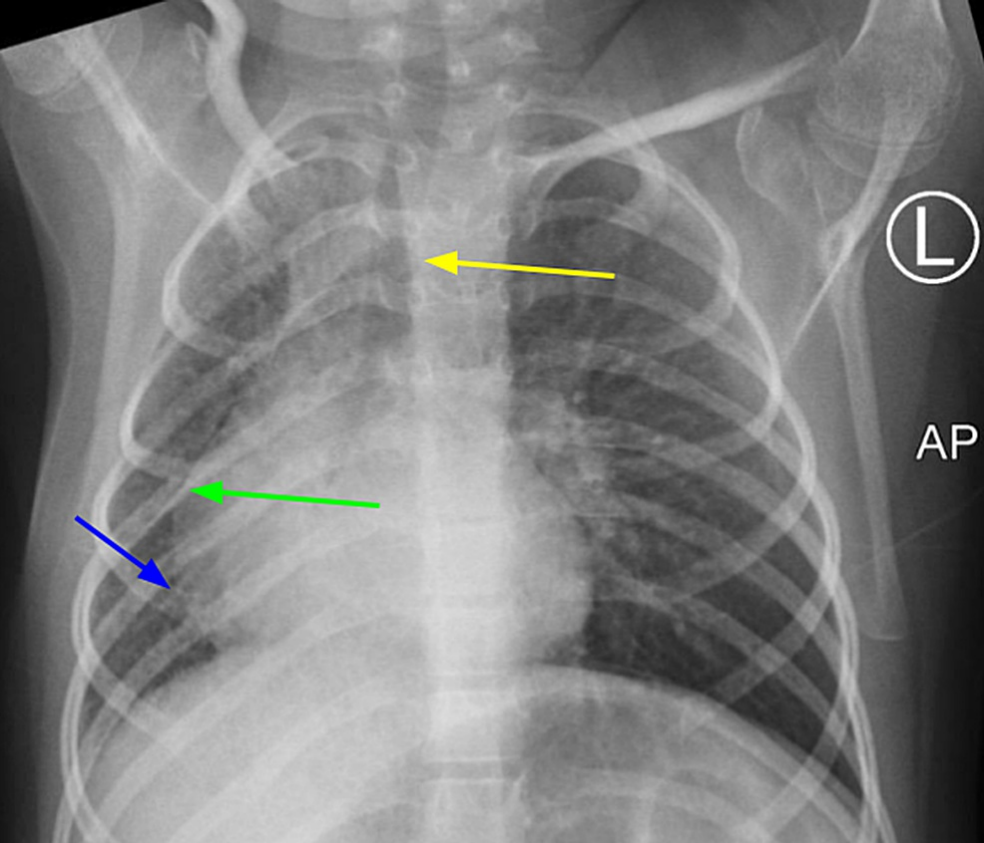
Anteroposterior chest x-ray (CXR) evaluating causes for chronic wet cough Anteroposterior (AP) CXR demonstrated nonspecific thickening of the interstitial markings more confluent at the right pulmonary field (blue arrow). Mediastinum was shifted to the right side (green arrow). The tracheal deviation was noted (yellow arrow).
